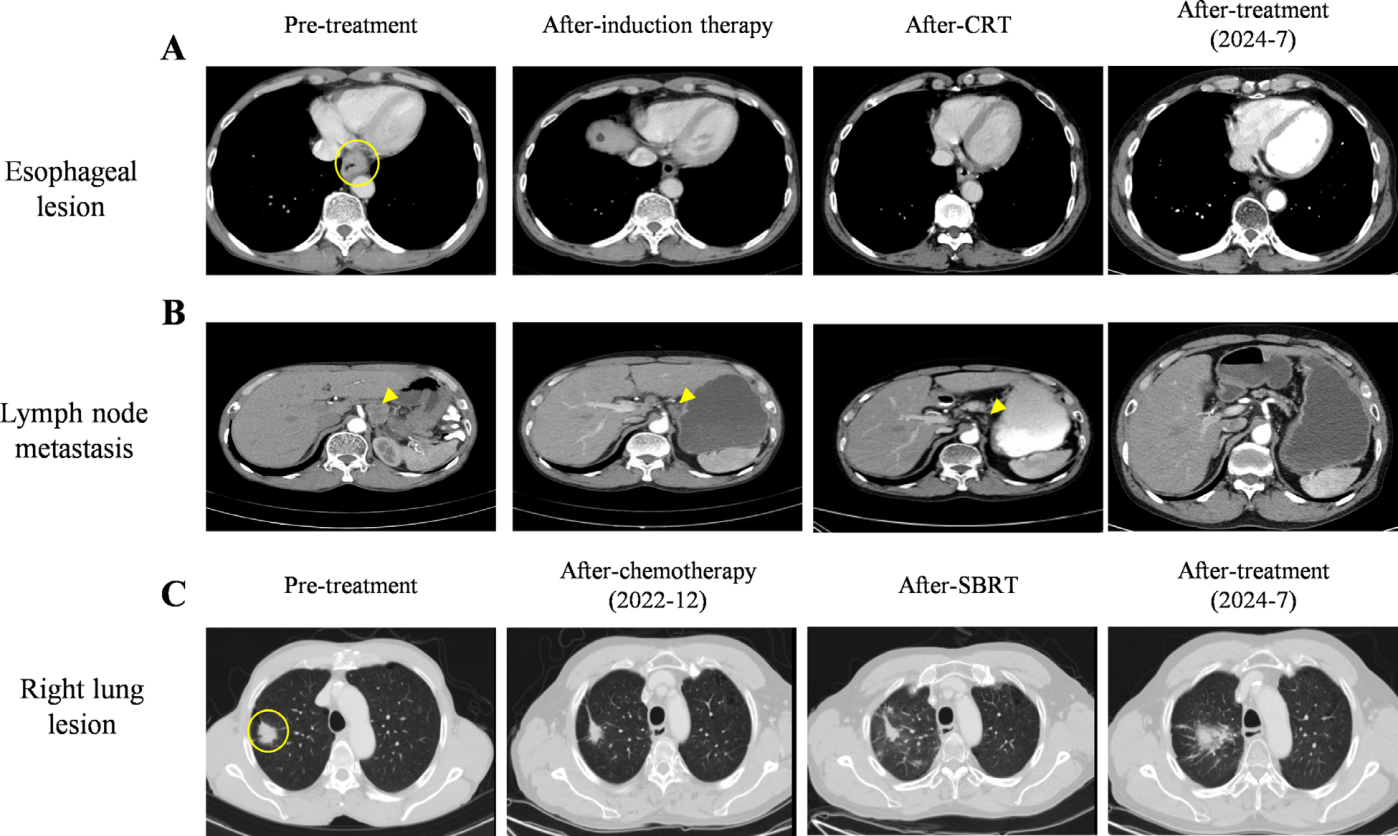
Images from pre- and after-treatment. (A) Pre- treatment, after-induction therapy, after-CRT, and after-treatment images of esophageal lesion on CT scans. The esophageal lesion showed a 10 mm thickened wall (○) on CT scan. (B) Pre- treatment, after-induction therapy, after-CRT, and after-treatment images of hepato-gastric space lymph node metastasis on CT scans. (C) Pre- treatment, after-chemotherapy, after-SBRT, and after-treatment images of lung lesion. Right lung lesion (29×19 mm) (○) on pre-treatment CT scan.
